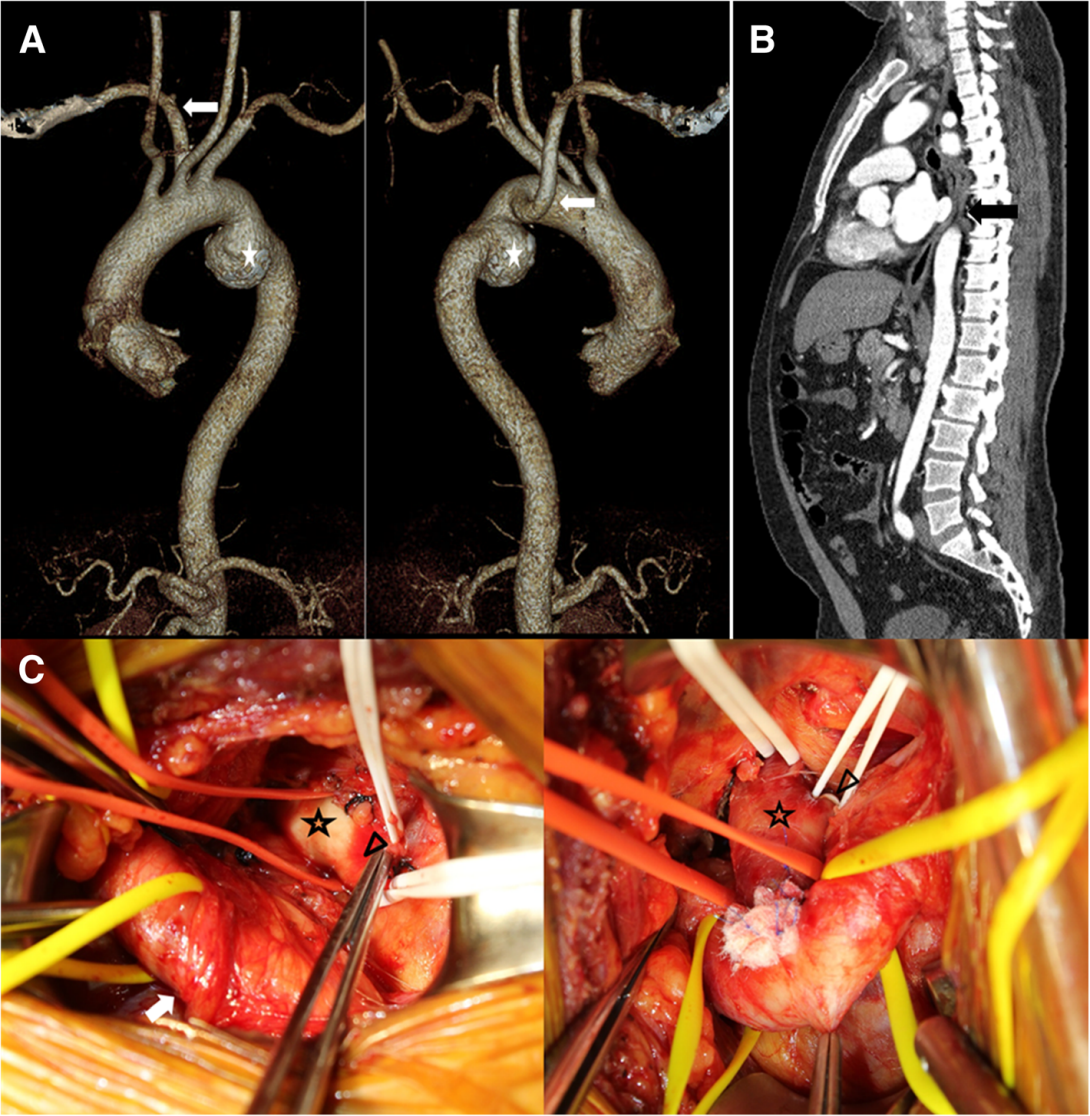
a-b Computed tomographic angiography showed a fusiform proximal descending aortic aneurysm (asterisk) distal to an ARSA (white arrow) with compressed esophagus (black arrow). c In operative field, ARSA (asterisk) to right carotid artery transposition (white arrow) with a ligation of the ARSA distal to the right vertebral (arrow head) and mammary arteries
